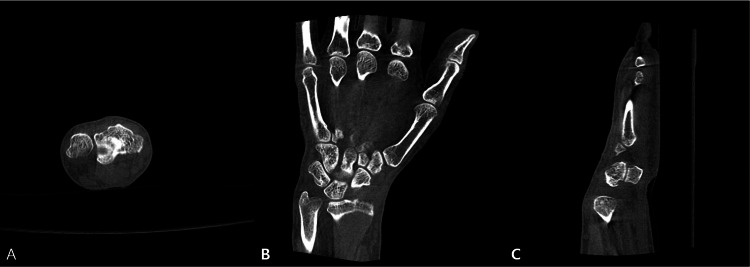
Computed tomography (CT) images of the wrist in the axial (A), coronal (B), and sagittal (C) planes demonstrate no acute fracture or other significant osseous abnormality.
